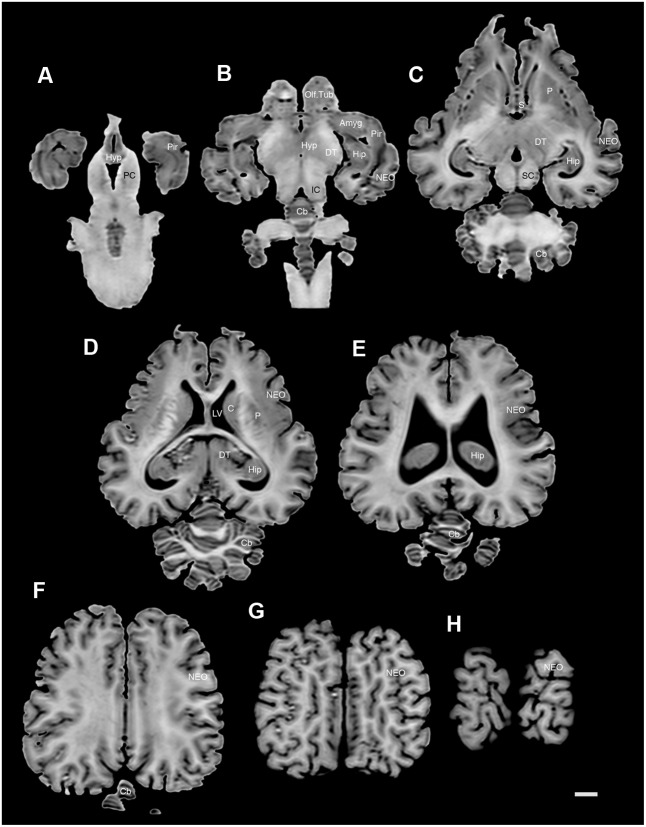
A series of horizontal structural MR images through the brain of the black rhinoceros (*D. bicornis*). (A) is the most ventral section and (H) is the most dorsal section, with each section having a thickness of 2 mm and each section being 6 mm apart. Note the typically mammalian topography of the various regions and structures of the brain. Scale bar = 1 cm. Amyg, amygdaloid body; C, caudate nucleus; Cb, cerebellum; DT, dorsal thalamus; Hip, hippocampus; Hyp, hypothalamus; IC, inferior colliculus; LV, lateral ventricle; NEO, neocortex; Olf. Tub, olfactory tubercle; P, putamen; PC, cerebral peduncle; Pir, piriform cortex; SC, superior colliculus.
